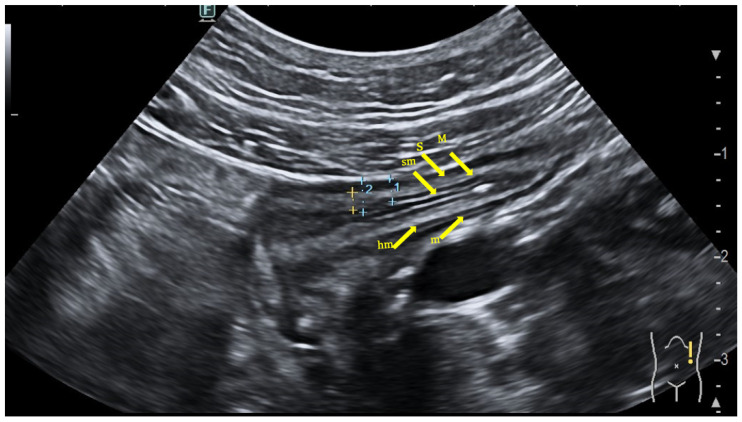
Bowel wall stratification at bowel ultrasound evaluation. hm: hyperechoic lumen interface of the mucosa; m: mucosa (hypoechoic); sm: submucosa (hyperechoic); M: muscolaris propria (hypoechoic); S: serosa (hyperechoic).
